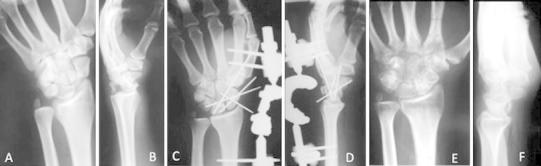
Posteroanterior (a) and lateral (b) radiographs of a 19-years-old male showing a trans-scaphoid trans-triquetral perilunate fracture- dislocation of the left wrist joint. Postoperative posteroanterior (c) and lateral (d) projections of the left wrist after reduction of the fracture dislocation and stabilization using an external fixator augmented with percutaneous K-wires. The scaphoid and the triquetral fractures were fixed with K-wires. e Posteroanterior and f lateral views of the same wrist 10 months postoperatively showing preservation of normal carpal alignment
